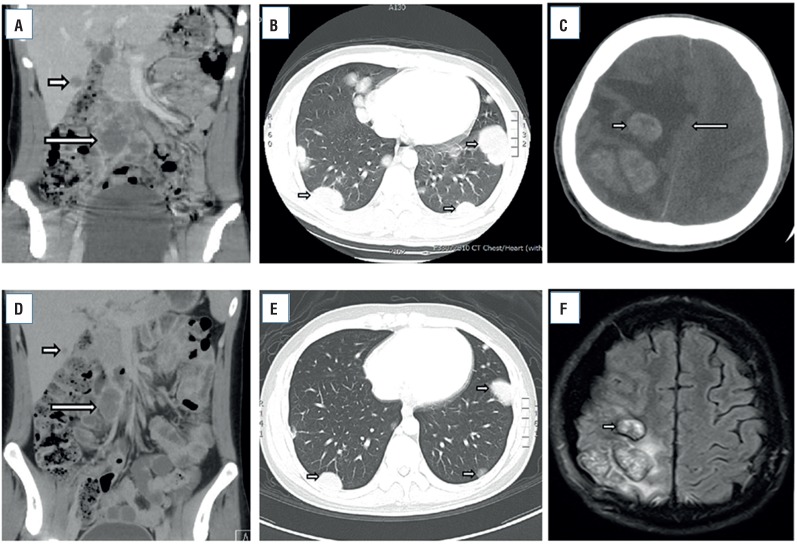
A) abdominal and pelvic CT showed liver (short arrow) and lymph node metastasis (long arrow) before chemotherapy treatment; B) chest CT showed multiple lung metastatic tu-mors before chemotherapy treatment (short arrows); C) Brain computed tomography (CT) sho-wed three heterogeneous, enhanced brain tumors with midline shift (short arrow: brain tumor, long arrow: midline shift); D) abdominal and pelvic CT showed shrinkage of the liver (short ar-row) and lymph node metastases (long arrow) after four courses of chemotherapy treatment; E) chest CT showed that multiple lung metastatic tumors had decreased in size after four courses of chemotherapy treatment (short arrows); F) brain MRI showed shrinkage (short arrow) and enhanced homogeneity of the brain tumor components without midline shift after completion of four courses of chemotherapy treatment.
